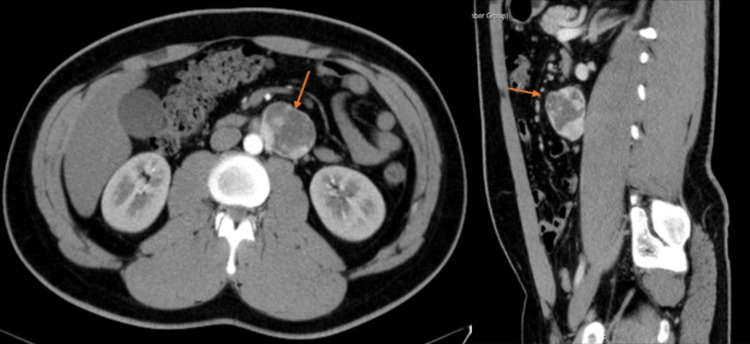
CT scan showing the retroperitoneal mass (arrow)
